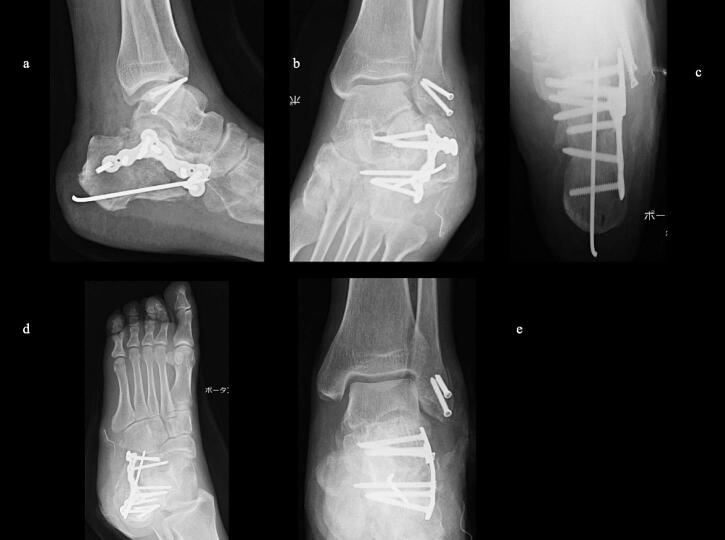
Simple X-ray after operation. a:Lateral view of the calcaneus. Calcaneal height and calcaneal length are reducted. b:Broden view of the calcaneus. Good congruity of the posterior talocalcaneal joint surfaces. c:Axial view of the calcaneus Lateral wall bulging was reduced. d:Oblique view of the foot. Good congruity of the calcaneocuboid articular surface. e:AP view of the ankle The lateral malleolus fracture was fixed with two cortical bone screws.
